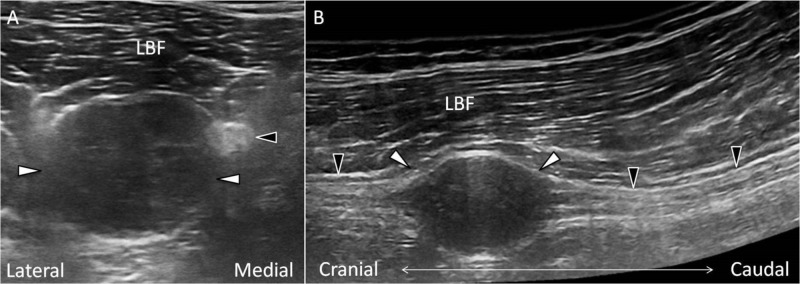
Ultrasonography of the sciatic schwannoma A tumor was noted beside the sciatic nerve with posterior enhancement in its short axis view (A). In the panoramic view, the tumor was noted below the long head of biceps femoris. The sciatic nerve bundles were seen at the cranial and caudal ends of the tumor (B). Black arrowheads: sciatic nerve; white arrowheads: schwannoma. LBF: long head of biceps femoris.
